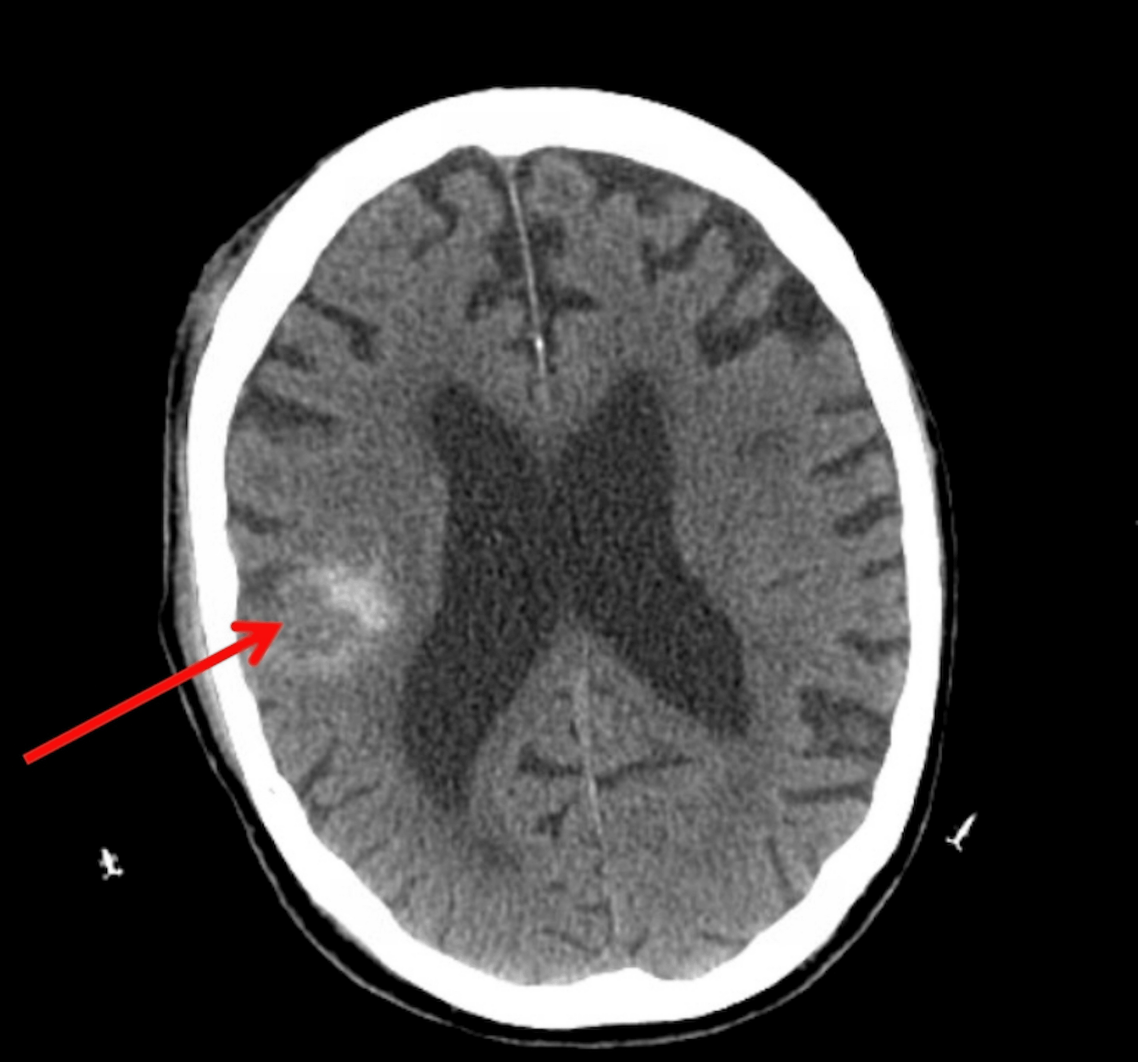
Repeat CT head of a 73-year-old male on clopidogrel demonstrating an intraparenchymal hemorrhage (arrow) CT: computed tomography
